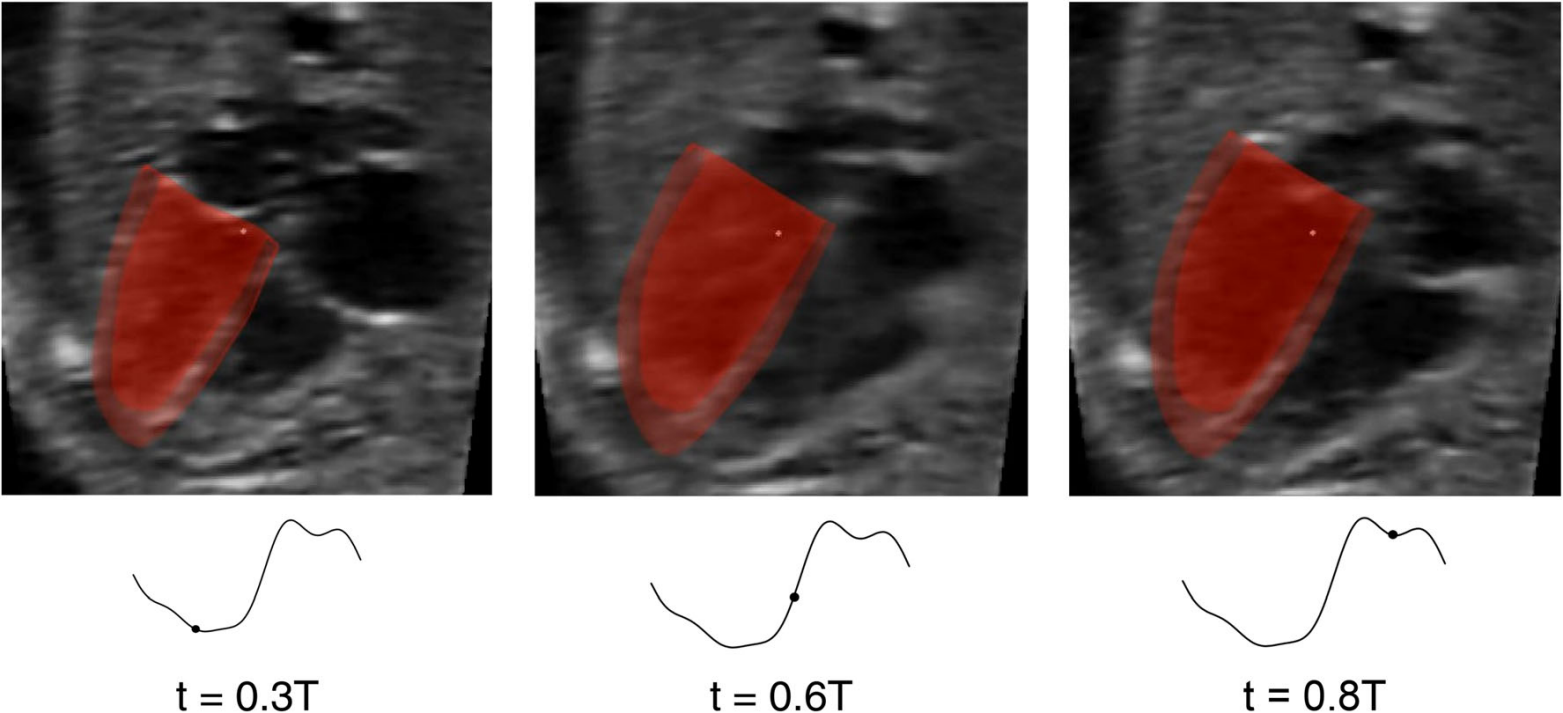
Three-dimensional fetal LV reconstructed from 4D ultrasound for a fetal heart at 22 weeks, with its motion tracked over the cardiac cycle, Here, t denotes time, while T denotes cardiac cycle duration.
